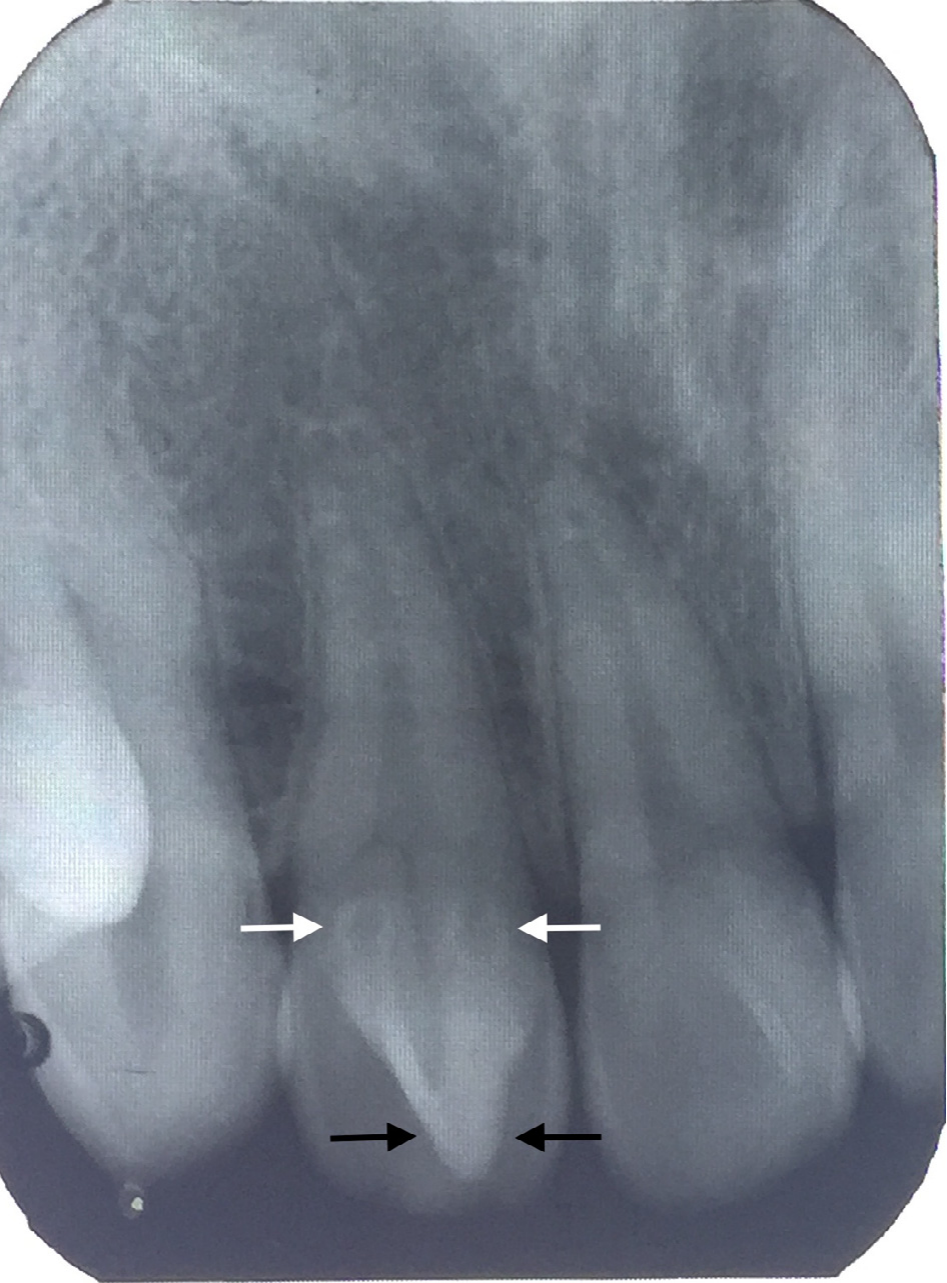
Intraoral periapical radiograph of right maxillary lateral incisor depicts talon cusp (black arrow) together with double dens invaginatus (white arrows).
